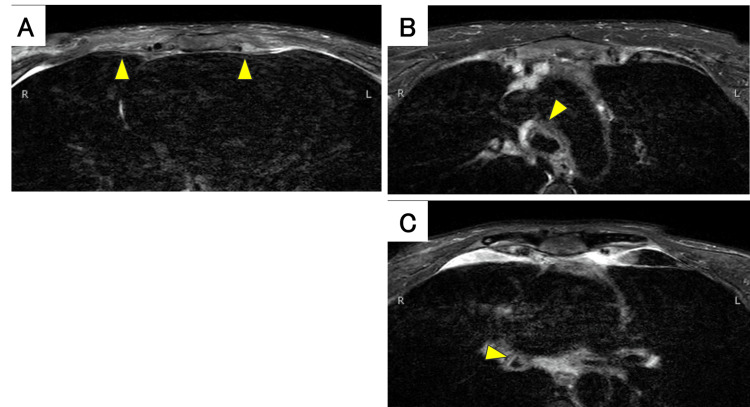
The MRI of the chest (A) High signal with unclear boundaries was observed in both parasternal regions, the anterior wall, and the intercostal region on the fat-suppressed T2-weighted images. (B, C) The thickening of the trachea and bronchial wall was also confirmed.
